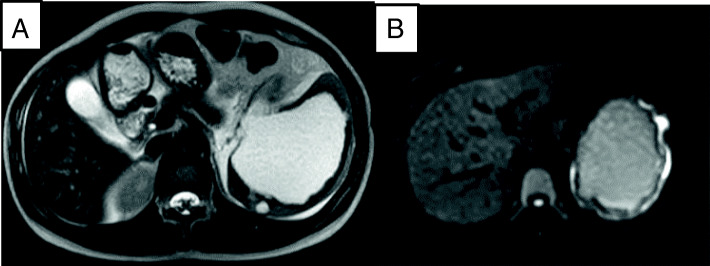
Contrast-enhanced MRI showed a cystic lesion, which exhibited slightly hyperintense signals on the T1- (a) and diffusion-weighted sequences and hyperintense signals on the T2-weighted sequence (b). No solid components or mural cysts were found in the cyst
